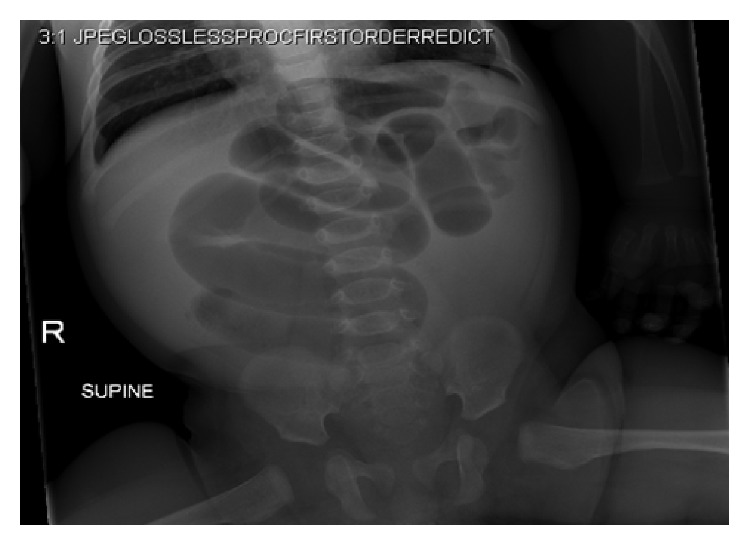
Supine abdominal plain film.
